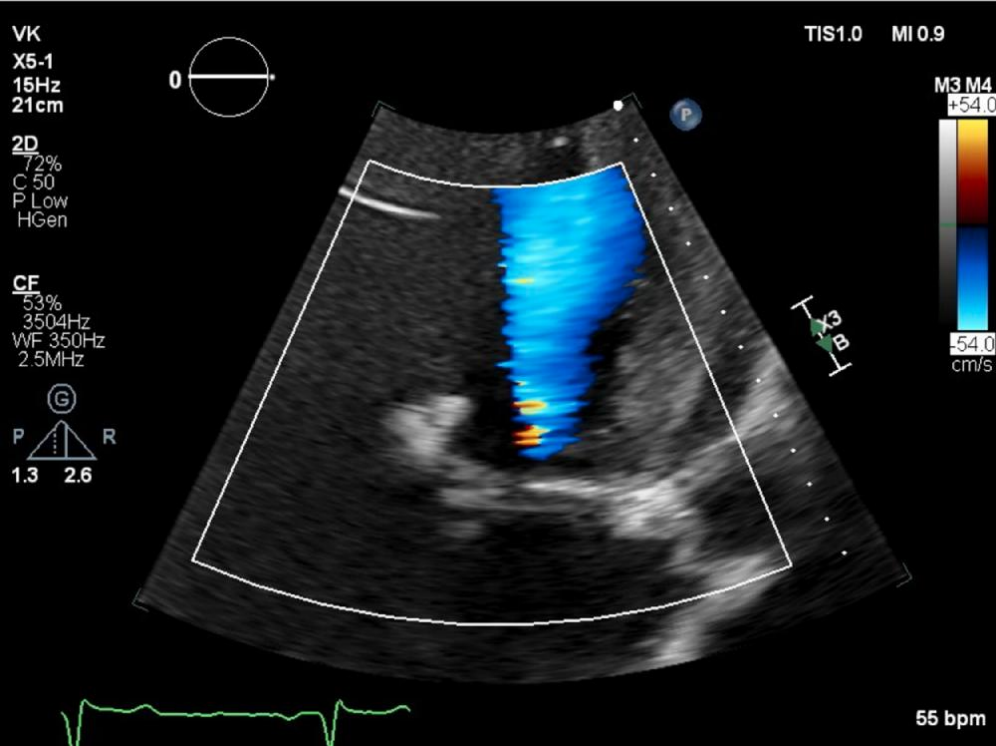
Transthoracic echocardiogram apical five-chamber view colour Doppler showing acoustic shadowing in the distal left ventricular outflow tract and aortic valve during systole.
